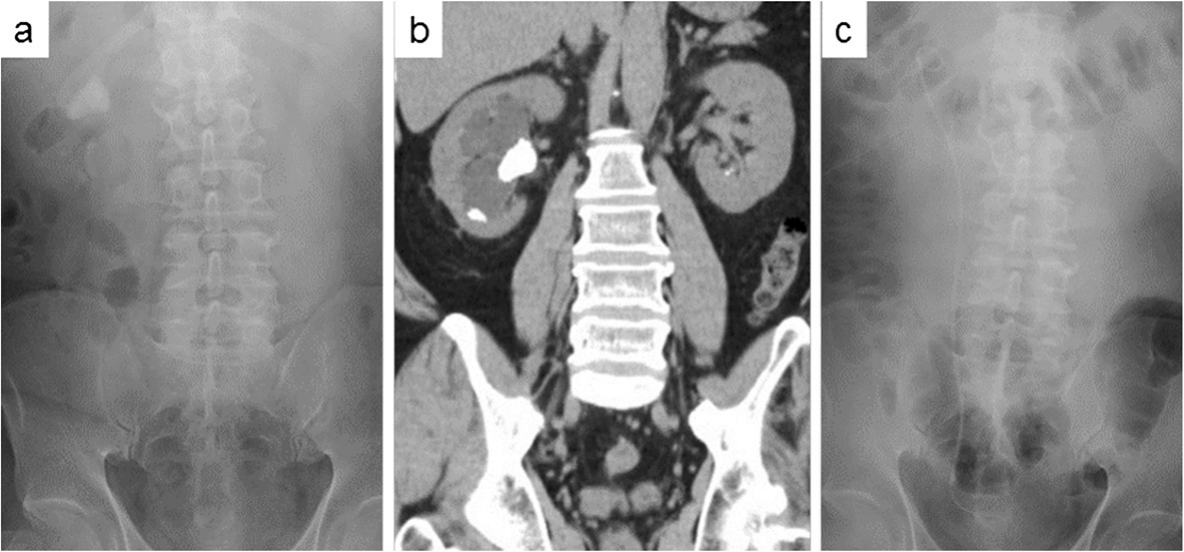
Pre- and post- operative images. (a) Preoperative kidney, ureter and bladder and (b) axial computed tomography. (c) Postoperative kidney, ureter and bladder.
